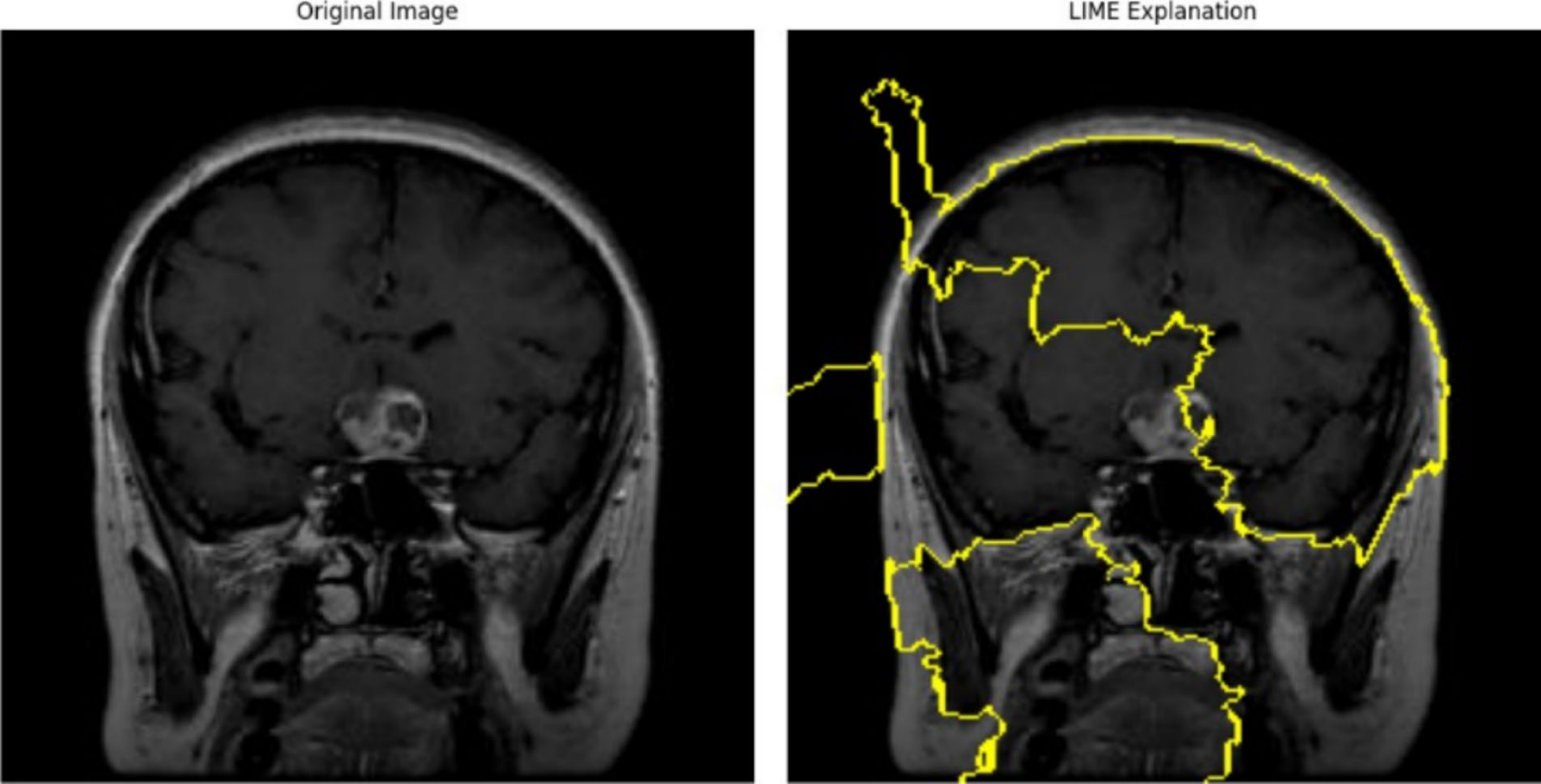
LIME visualization of pituitary MRI image in early stages of Xception model.
